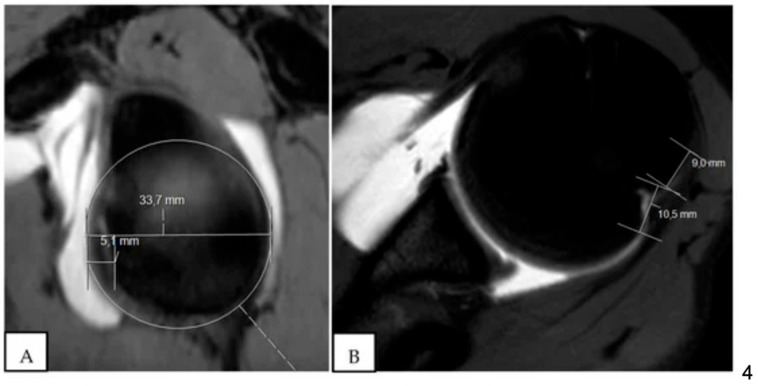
On-track lesion on magnetic resonance imaging (MRI) arthrography. (A) In this case, the glenoid track is equal to 23 mm (33 − 5 × 0.83 = 23). (B) The Hill–Sachs index (HSI) is 19.5 mm (10.5 + 9). It is an on-track lesion because the glenoid track (23 mm) is greater than the HSI (19.5 mm) [16].
